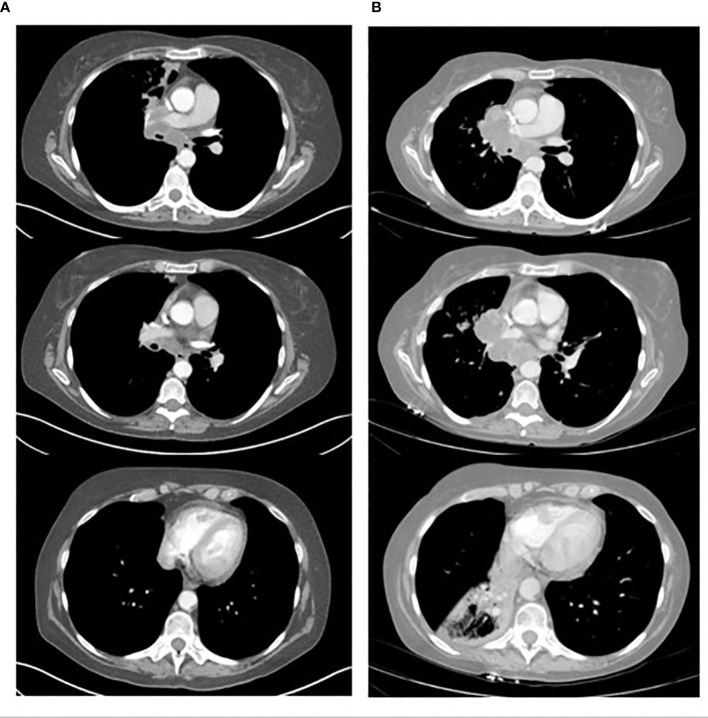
Shows the CT scans of a 61-year-old patient with NSCLC. (A) shows 3 axial CT scan images of the tumor located in the right para-mediastinal area and lymph node enlargement at level 7 and 10. (B) corresponding axial CT scan images after 2 cycles of Pembrolizumab monotheraphy (49 days later) showing clear increase in tumor mass and lymph nodes at level 7 and 10 and atelectasis of right lower lobe.
